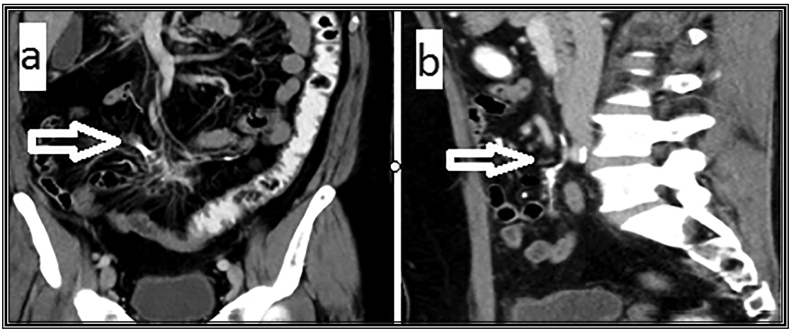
Abdominal computerized tomography (CT) with the contrast. (a) Coronal view of the mesentery shows diffuse focal fat opacification of the mesentery with intervening dense calcified densities (white arrow). (b) Sagittal view shows very thin dense shadows appear longitudinal in position (white arrow) with surrounding mesenteric focal stranding opacity at the site related to previous operations.
